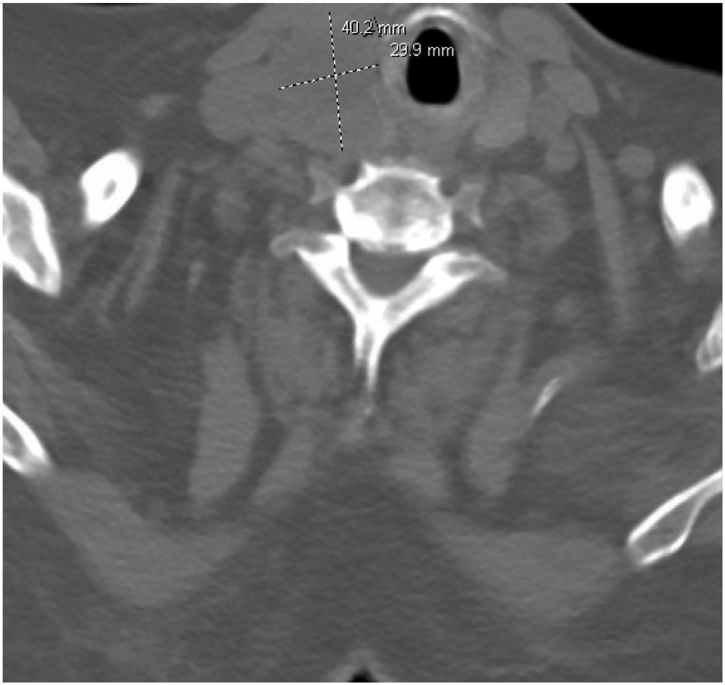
Computed tomography (CT) scan showing the esophageal adenocarcinoma.
